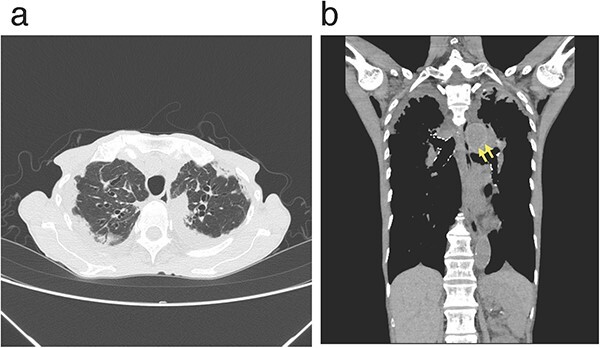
Chest computed tomography images displaying pleural thickening with pulmonary fibrosis, volume loss, and traction bronchiectasis in the bilateral upper lobes (a, transverse view of lung window image; b, coronal view of mediastinal window image). Note that the aortic arch and left pulmonary artery are in close contact (*arrows*).
